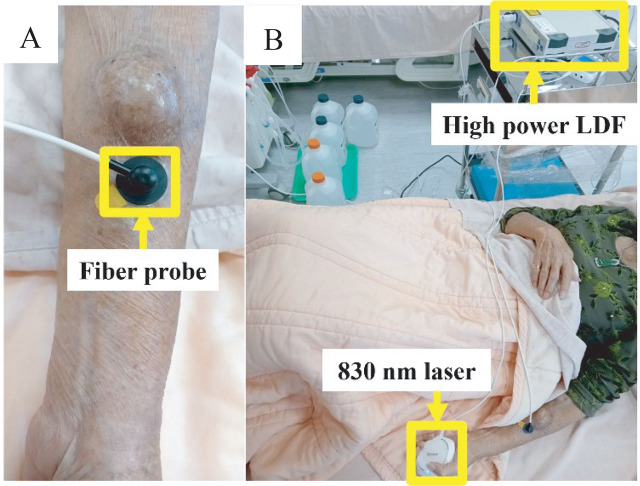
(A) The fiber probe of the high-power LDF was placed at the edge of the AVF. (B) The actual tested situation of HD patients receiving 830 nm laser on the palm. AVF, arteriovenous fistula; HD, hemodialysis; LDF, laser Doppler flowmetry.
